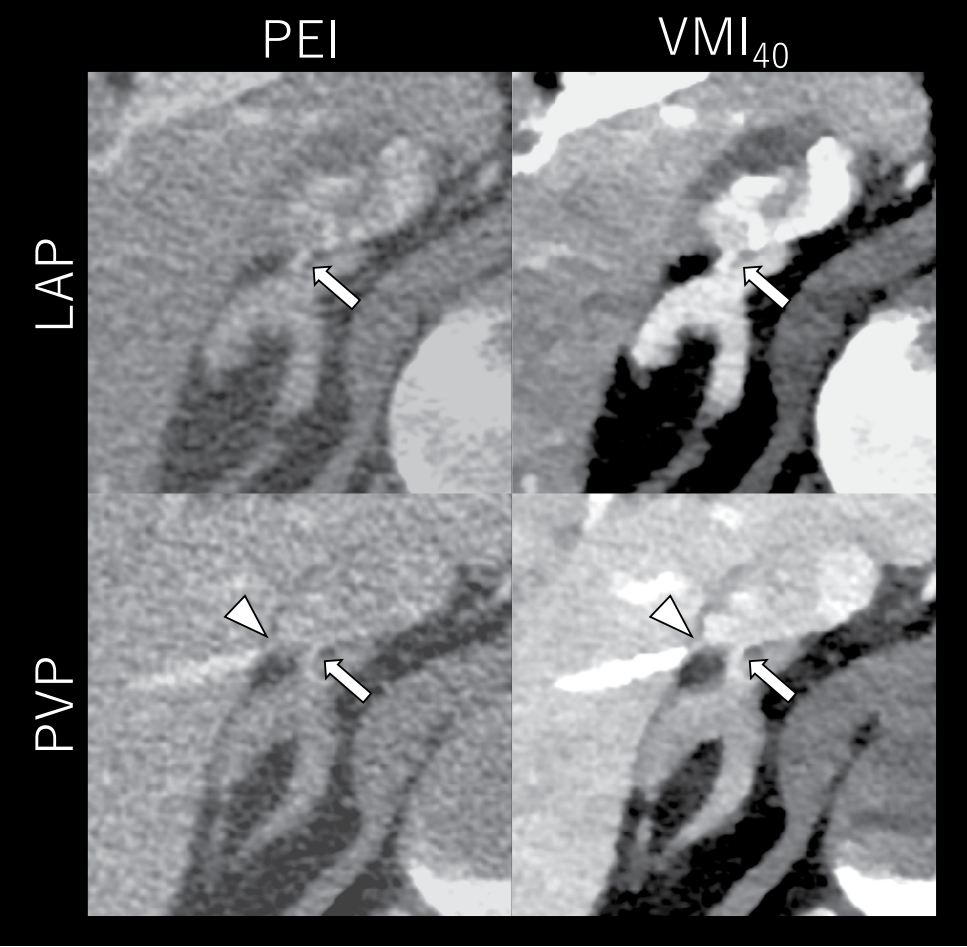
CT images of a 44-year-old male with bilateral PA (BMI: 24.4 kg/m^2^). The upper and lower rows display late arterial phase (LAP) and portal venous phase (PVP) images, respectively, reconstructed with polyenergetic images (PEI) and virtual monoenergetic images at 40 keV (VMI_40_). The window display is optimized for each image series.VMI_40_ at PVP most clearly delineated the relationship between the RAV (arrows) and accessory hepatic vein (arrowheads), both independently draining into the inferior vena cava, while also providing adequate RAV visibility
